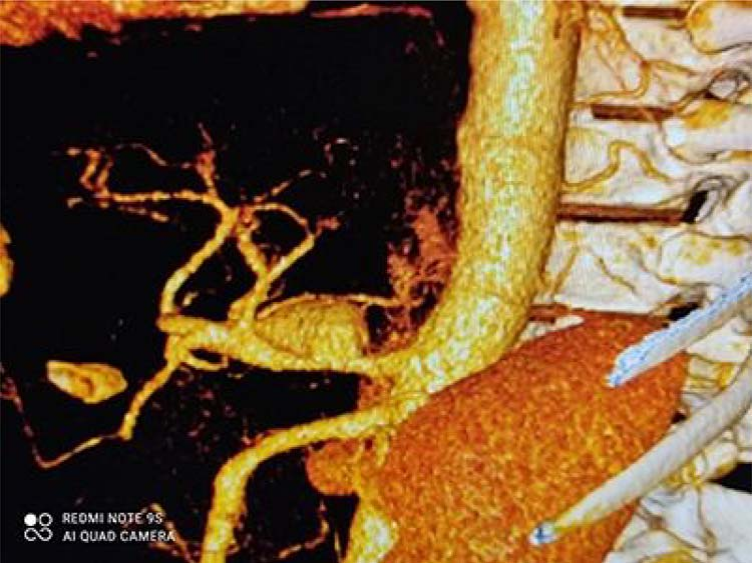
3D dimensional CT angiography shows CAA before intervention.
